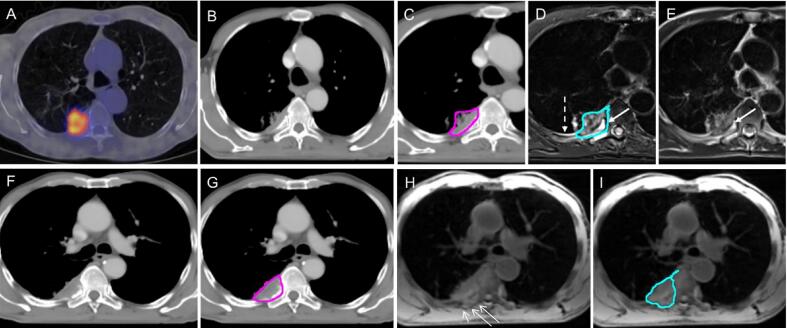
Chest wall invasion; (A) PET-CT scan, (B) CT scan in mediastinal windows (L: 46, W: 600 HU) showing no obvious cortical destruction of bone (C) CT scan with CT consensus contour (pink), (D) T_2_w TSE Dixon Water with hyperintensity of the rib (arrow). The MR consensus contour (light blue) reflects the choice of the clinicians to include the posterior rib in the GTV. There is also pleural reaction extending along the chest wall (broken arrow) and not included in the contour, (E) T_2_w TSE non-fat-sat image showing hyperintensity of the rib (arrow), (F) CT scan now showing cortical invasion, (G) CT scan showing inclusion of the involved bone within the CT consensus contour (pink), (H) T_1_w Radial GRE showing deeper invasion into posterior structures (thin arrows), (I) Fat-suppressed T_1_w Radial GRE with MR consensus contour (light blue) demonstrating slightly more generous volume of the MR consensus contour posteriorly. Although on the same slice on CT (E and G), cortical involvement was evident, and hence appropriately included in the GTV the MR images suggested deeper invasion included in the MR consensus contour. (For interpretation of the references to colour in this figure legend, the reader is referred to the web version of this article.)
